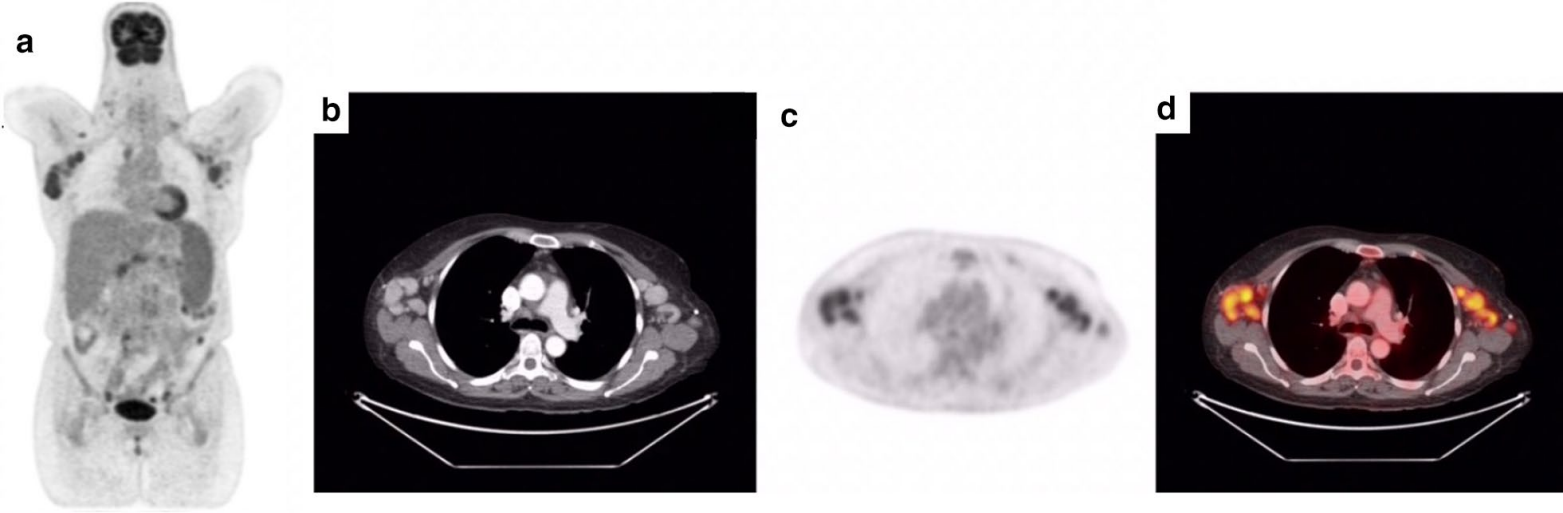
a displays the PET scan of a subject with multicentric Castleman disease (MCD) in the coronal view. Enlarged axillary and cervical lymph nodes and an enlarged spleen are seen in a, demonstrating the systemic nature of MCD. b–d display the CT, PET, and PET/CT cross-sectional scans of the subject’s thorax, respectively. d displays high uptake of lymph nodes in the right and left axillary regions as shown in a
